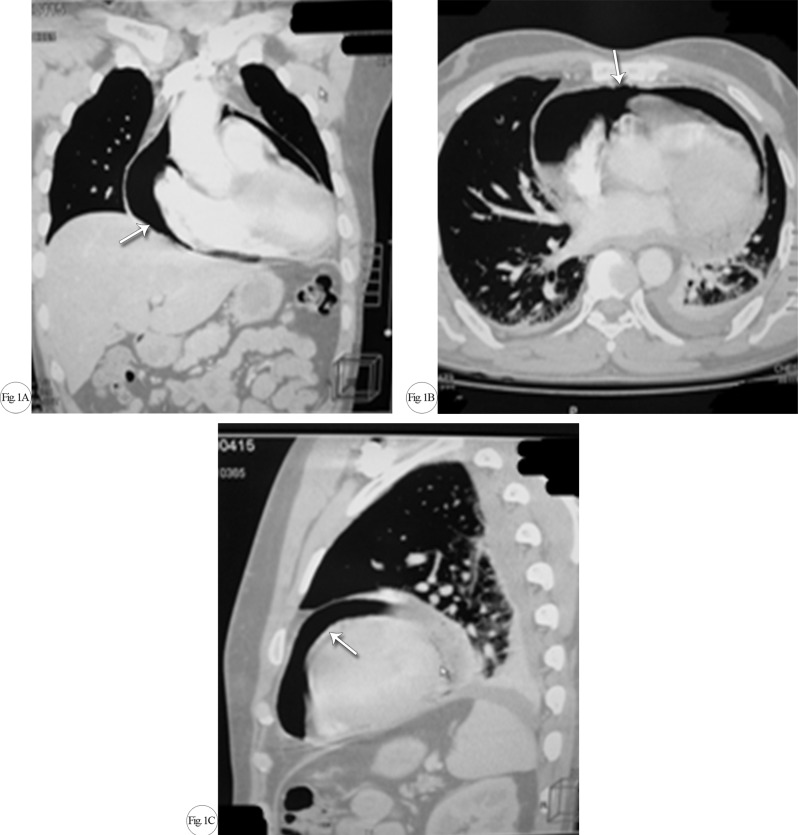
Given the CT scan findings, the patient was admitted to the CCU for observation with continuous cardiac and hemodynamic monitoring. Urgent bedside echocardiography was done, and it ruled out tension pneumopericardium. Chest X-ray was performed to evaluate the extent of the pneumopericardium and provide reference for follow-up (Figure 2).
